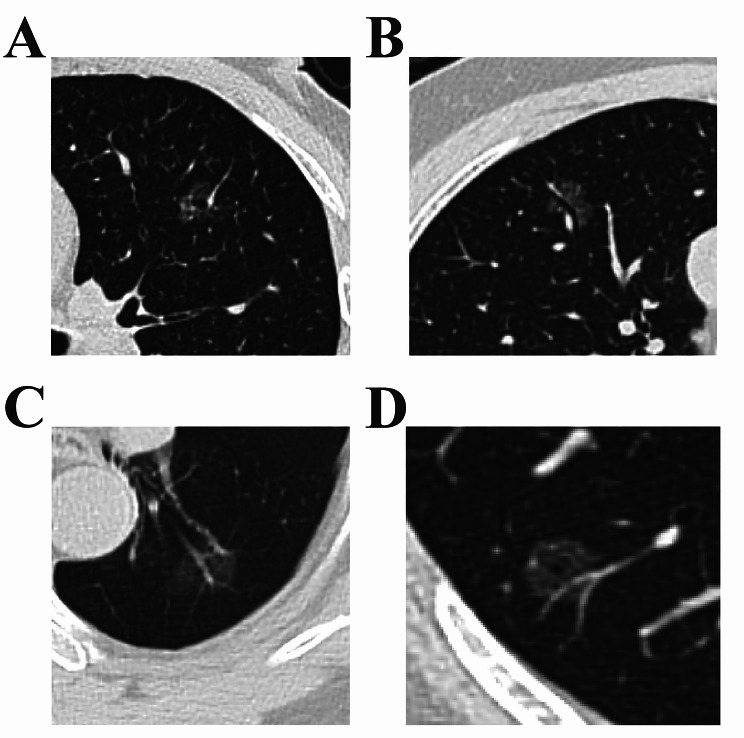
Image features of malignant GGNs in Axial HRCT. (A) Adenocarcinoma in a 55-year-old male. HRCT shows a 15 mm focal ground-glass nodule with vacuole sign sign and intra-nodular vessel sign in the left upper lobe. (B) Adenocarcinoma in a 57-year-old female. HRCT shows a 15 mm focal ground-glass nodule with air bronchogram sign and intra-nodular vessel sign in the right upper lobe. (C) Adenocarcinoma in a 67-year-old male. HRCT shows a 18 mm focal ground-glass nodule with intra-nodular vessel sign in the left lower lobe. (D) Adenocarcinoma in a 60-year-old male. HRCT shows a 18 mm focal ground-glass nodule with vacuole sign sign and intra-nodular vessel sign in the right lower lobe
